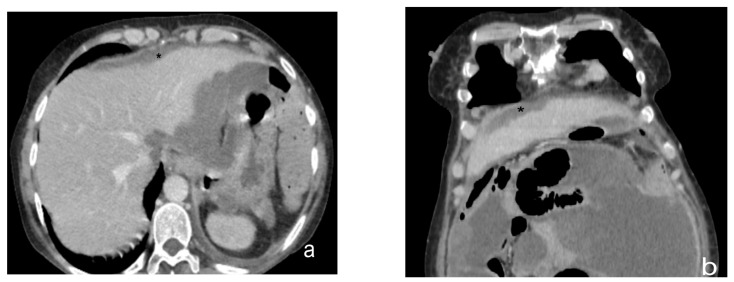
Contrast CT scan, axial view (a) and coronal view (b). Case of a plaque-like pattern in peritoneal carcinomatosis. Peritoneal thickening (black star) located on the lower surface of the right diaphragm, depressing the liver surface.
